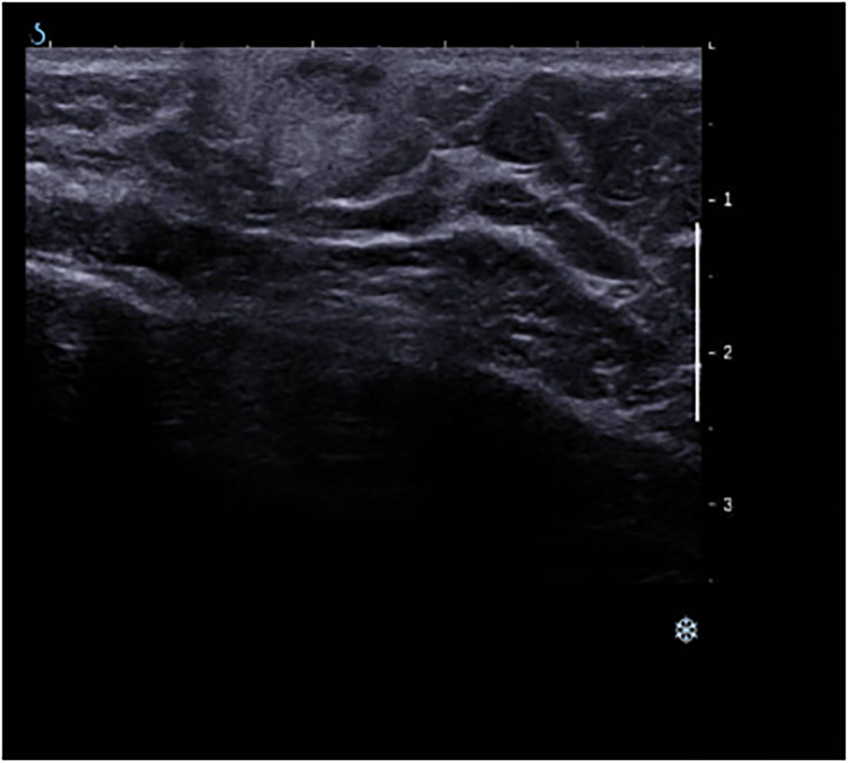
B-mode ultrasound demonstrates superficial circumscribed hypoechoic oval masses with parallel orientation within the skin with associated hyperechoic rim in keeping with an infected sebaceous cyst.
